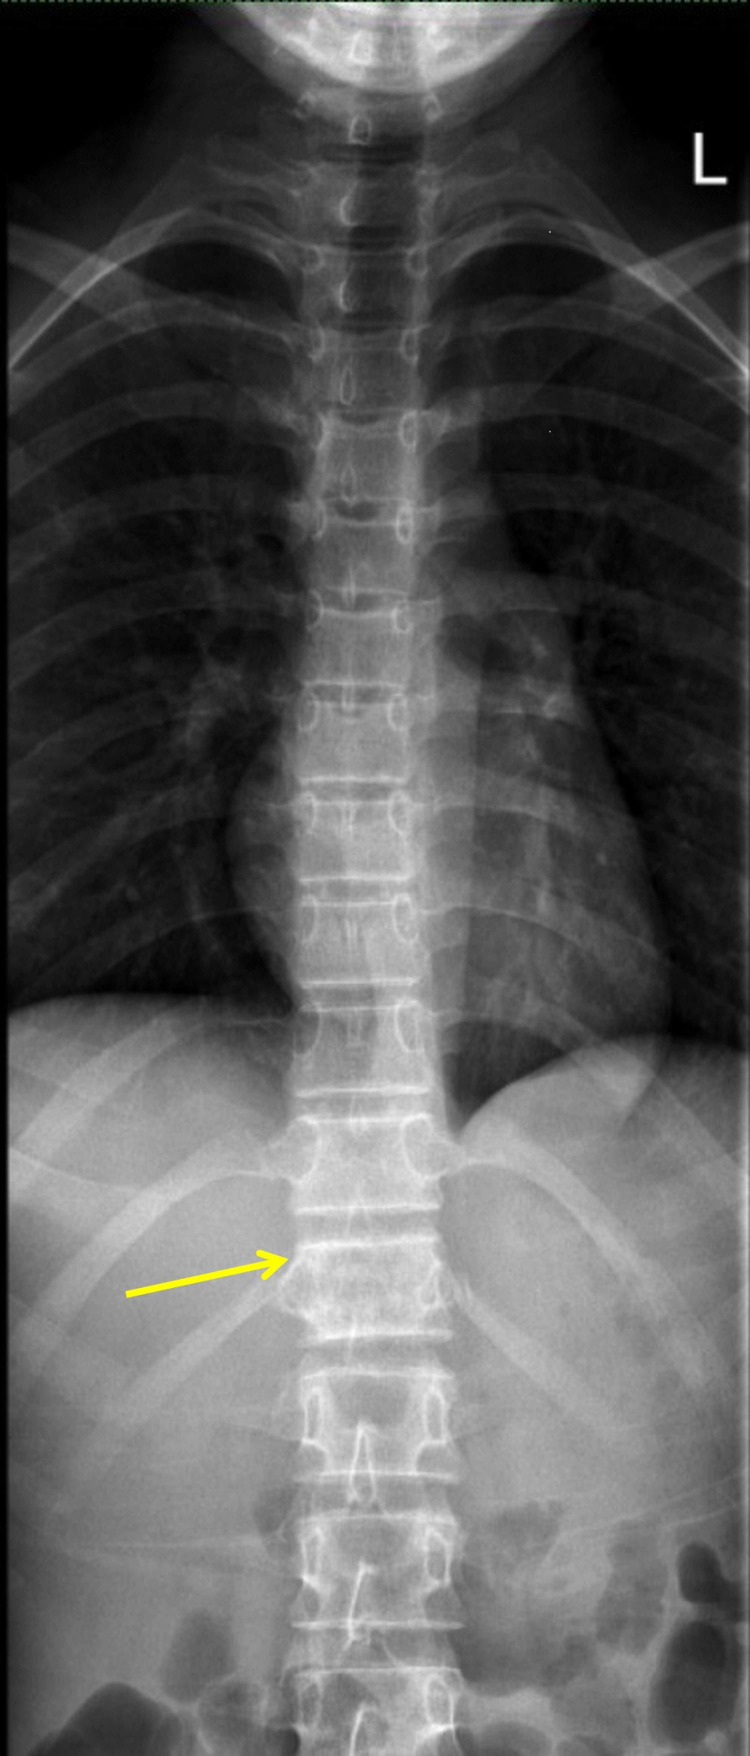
Pre-operative plain radiograph of the spine (anteroposterior view). The arrow shows a mixed sclerotic bony lesion of the T12 vertebral body with preserved vertebral height.
